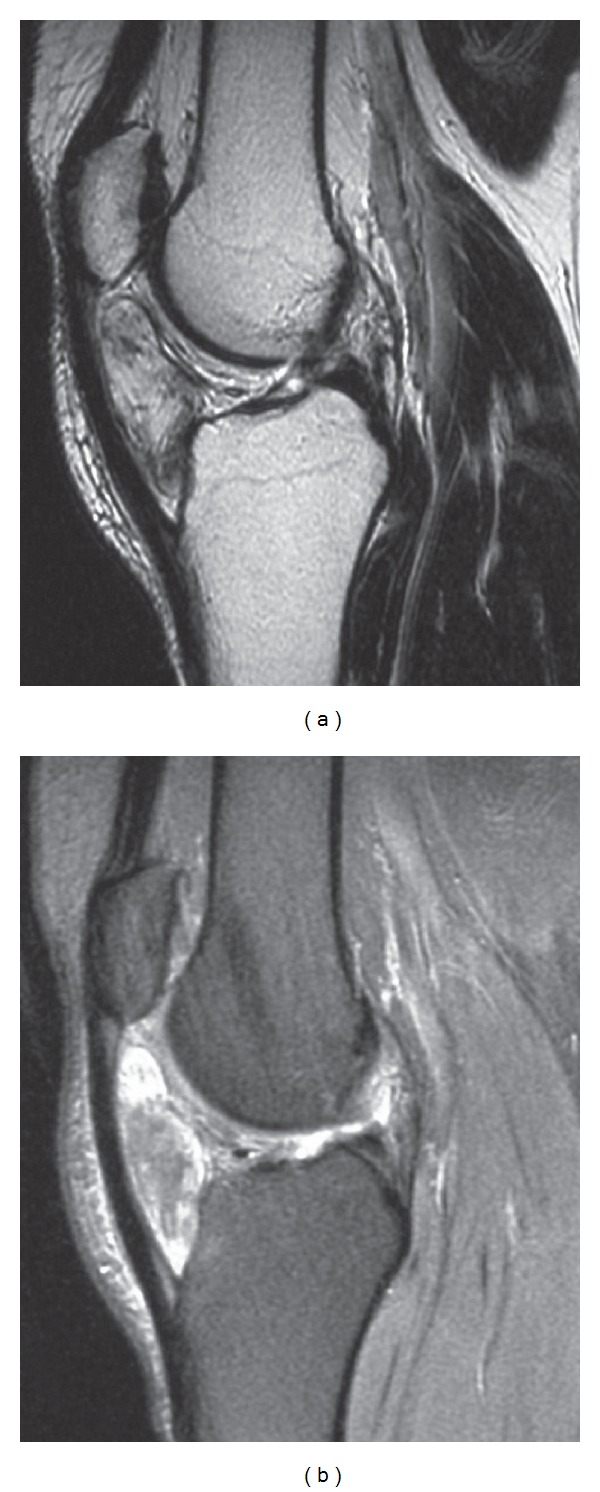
(a) Axial T1-weighted image. (b) Axial T2-weighted image showing clearly the mass in Hoffa's fat pad underlying the patellar tendon and extra-articular.
